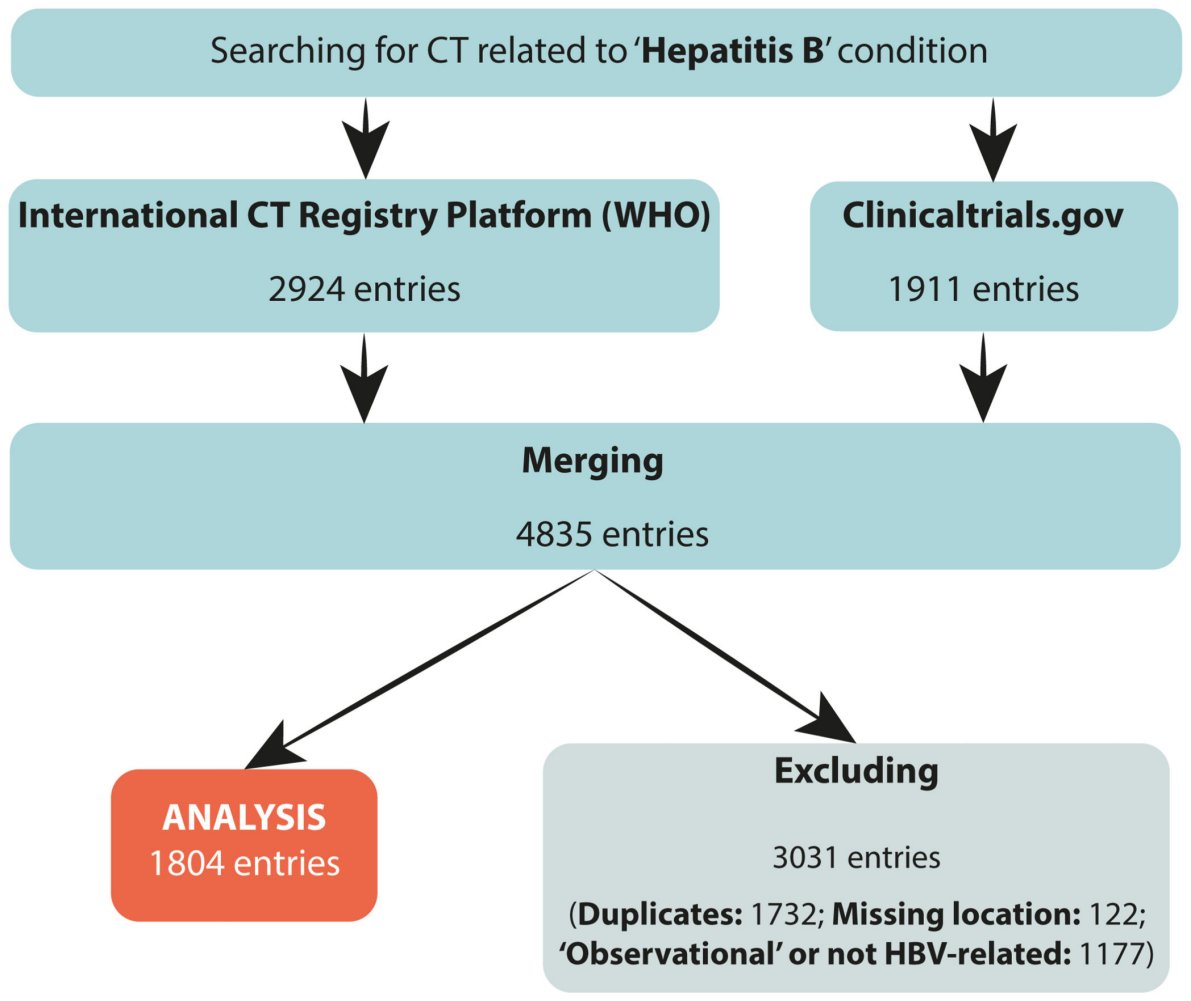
Protocol and decision tree associated with investigation of HBV Clinical Trials (CT). The International CT Registry Platform (ICTRP) from the World Health Organization (WHO), and ‘ClinicalTrials.gov’ registries were searched. Search criteria were ‘Hepatitis B’. Data from each registry were merged, and CT were excluded to remove (1) duplicates, (2) studies with missing location data or (3) those that did not meet criteria for HBV-related interventional CT, (i.e: studies categorized as ‘observational’ or patients were not infected with HBV or were not part of the evaluation of an antiviral drug against HBV). The material is presented according to the standardized PRISMA reporting criteria.
